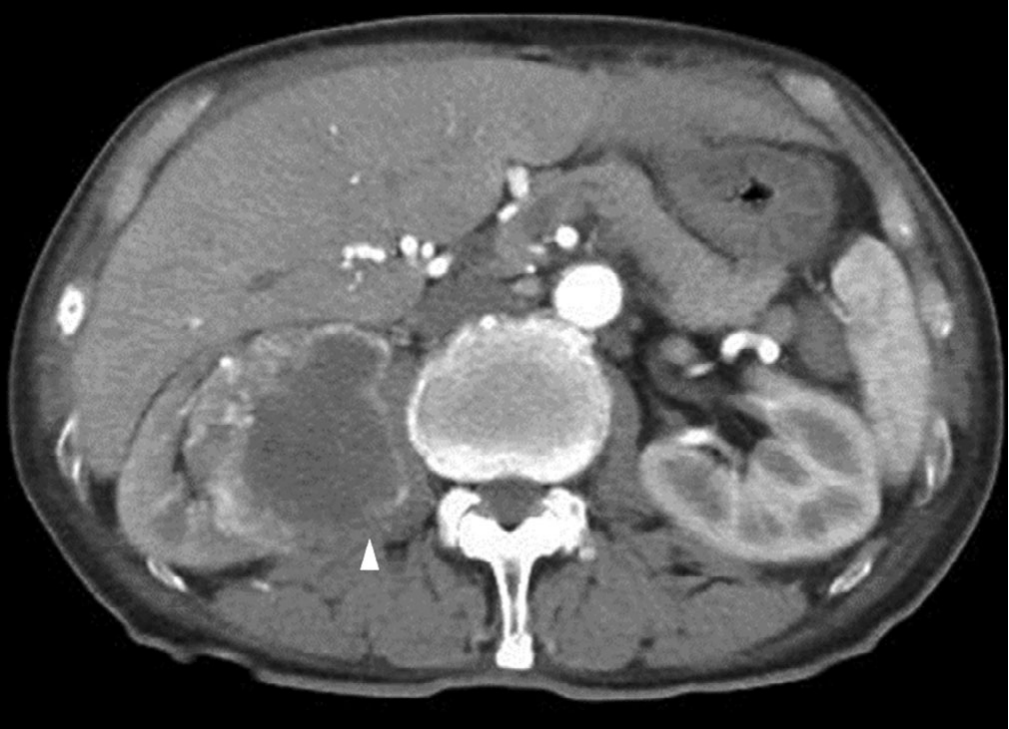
Abdominal computed tomography showing a right renal tumor in the lower pole with suspected invasion to the right iliopsoas muscle (arrow)
